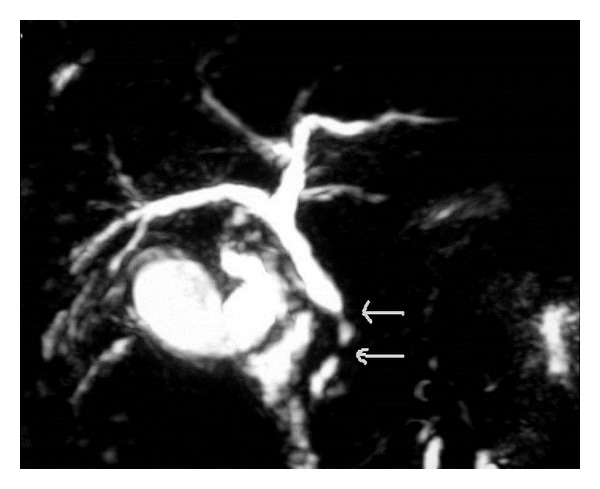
MRI cholangiopancreatography: wall regular thickening of the main bile duct with distal stenoses.
